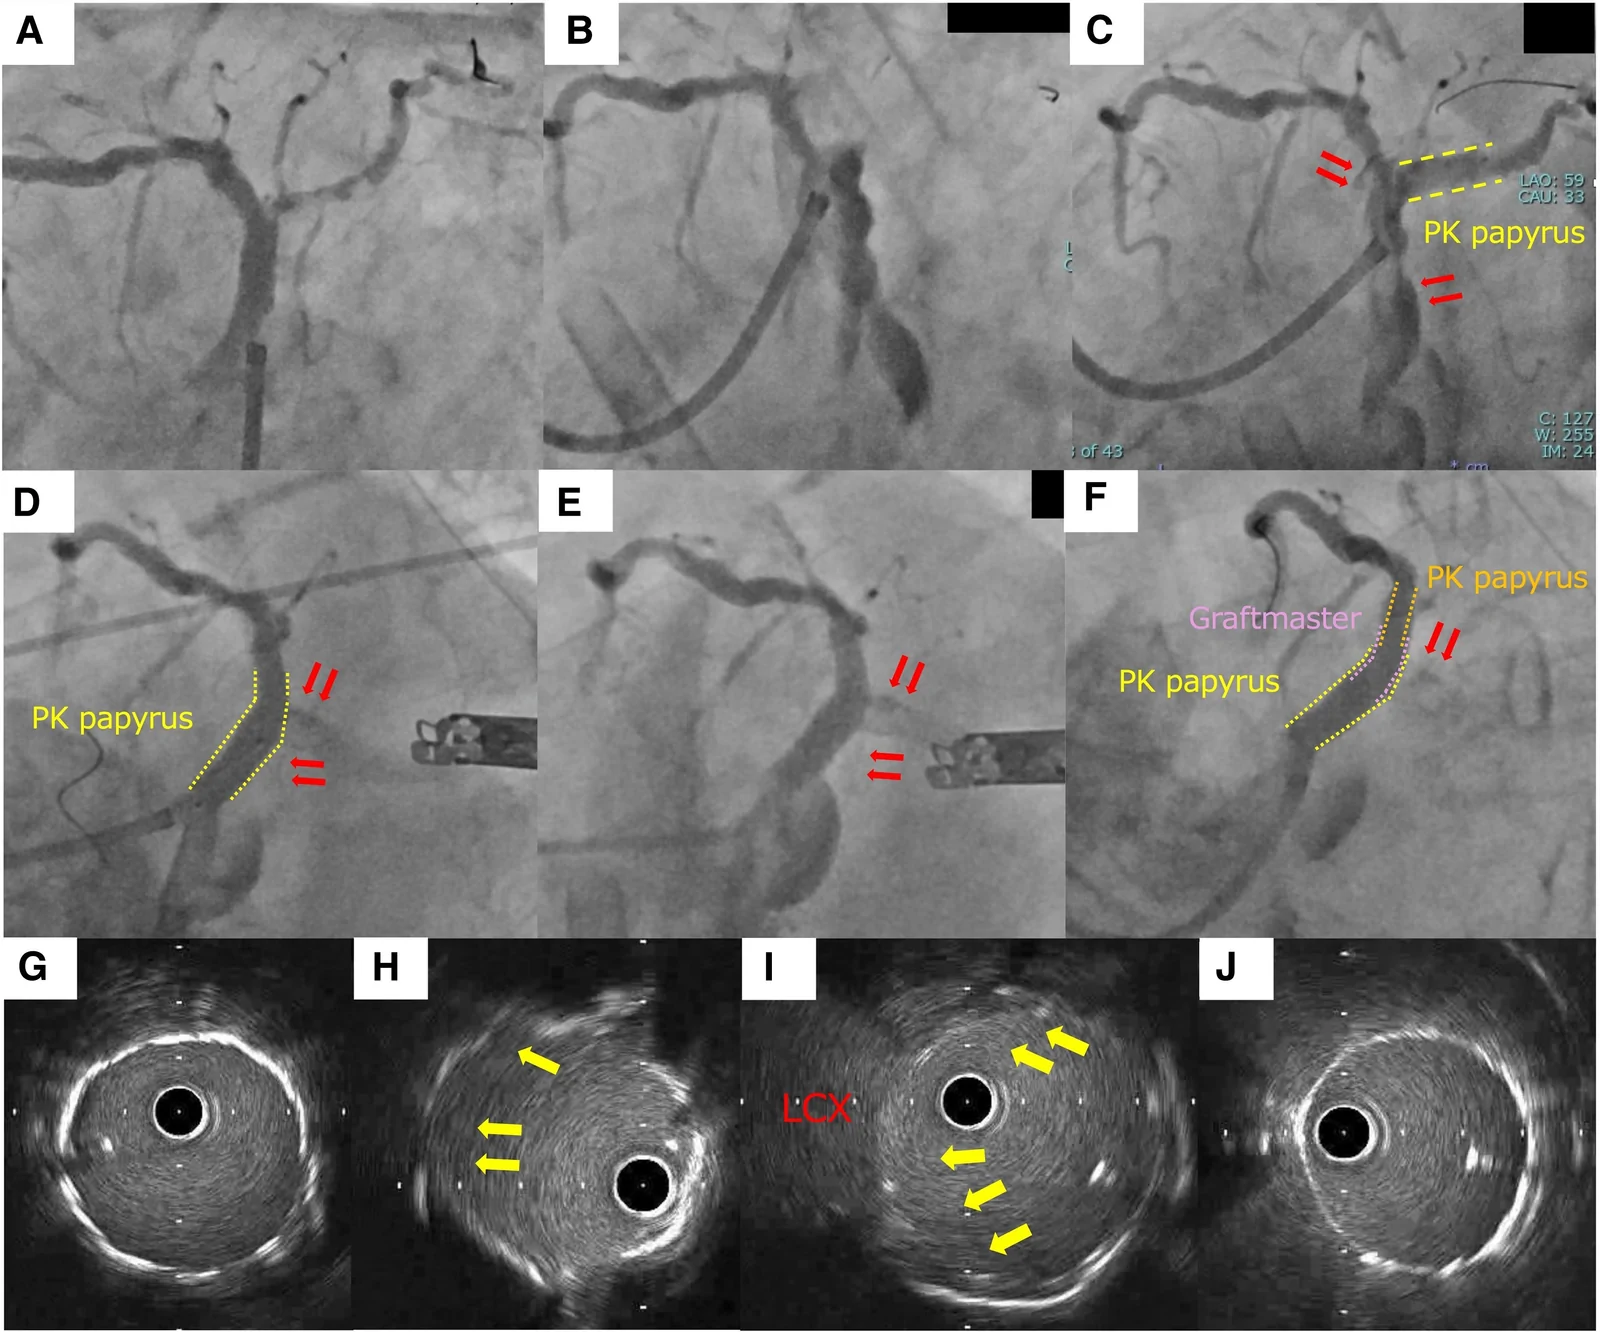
Serial angiographic and intravascular ultrasound findings. (*A*) Initial angiography. (*B*) Coronary perforation occurred at the left circumflex artery ostium. (*C*) Deployment of a PK Papyrus (3.5 × 20 mm) at the left circumflex artery ostium. (*D*) Additional deployment of a PK Papyrus (3.5 × 20 mm) from the left main trunk to the left anterior descending artery; however, contrast extravasation persisted. (*E*) Persistent contrast extravasation despite repeated post-dilatation using a 5 mm non-compliant balloon and a 1-h waiting period. (*F*) Additional covered stenting with a Graftmaster (3.5 × 16 mm) and a PK Papyrus (2.5 × 20 mm) failed to achieve complete sealing of the perforation. (*G*) Intravascular ultrasound image immediately after PK Papyrus deployment. Intravascular ultrasound imaging was performed using the AltaView system (Terumo, Tokyo, Japan) at 60 MHz with a pullback speed of 9 mm/s. The gain settings were G 15, C 3, Gm 7, S 1, and T 3. (*H*) Intravascular ultrasound image after post-dilatation using a 5 mm non-compliant balloon. (*I*) Intravascular ultrasound image after repeated post-dilatation and a 1-h waiting period. (*J*) Intravascular ultrasound image after Graftmaster deployment. Red arrows indicate contrast extravasation. The yellow arrow indicates an area of reduced conspicuity of the Papyrus membrane.
